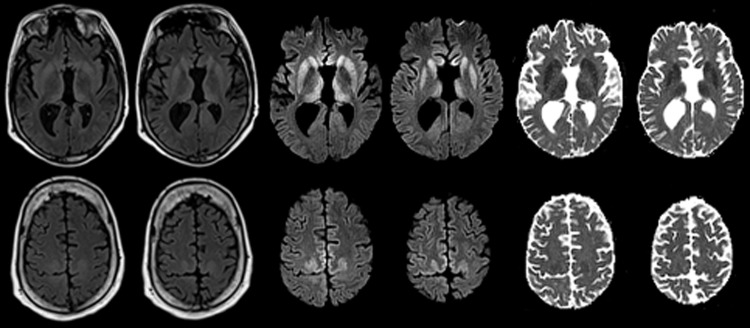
Maps showing axial fluid attenuated inversion recovery (FLAIR), diffusion-weighted imaging (DWI), and apparent diffusion coefficient (ADC) at the level of the basal nuclei (top row) and dorsal frontoparietal cortex (bottom row) of the brain of a 33.8-year-old man with agenesis of the corpus callosum, schizencephaly, and heterotopia. Note the symmetrical DWI signal hyperintensities in the striatum and dorsomedial part of the thalami. In addition, DWI signal hyperintensities occurred in the cingulate, precuneus and in the dysplastic gray matter along the anterior lips of the schizencephalic clefts at the level of the precentral gyri. The signal abnormalities are associated with decreased diffusivity on ADC maps and are much less prominent on FLAIR images. These findings are highly suggestive of Creutzfeldt-Jakob disease.
